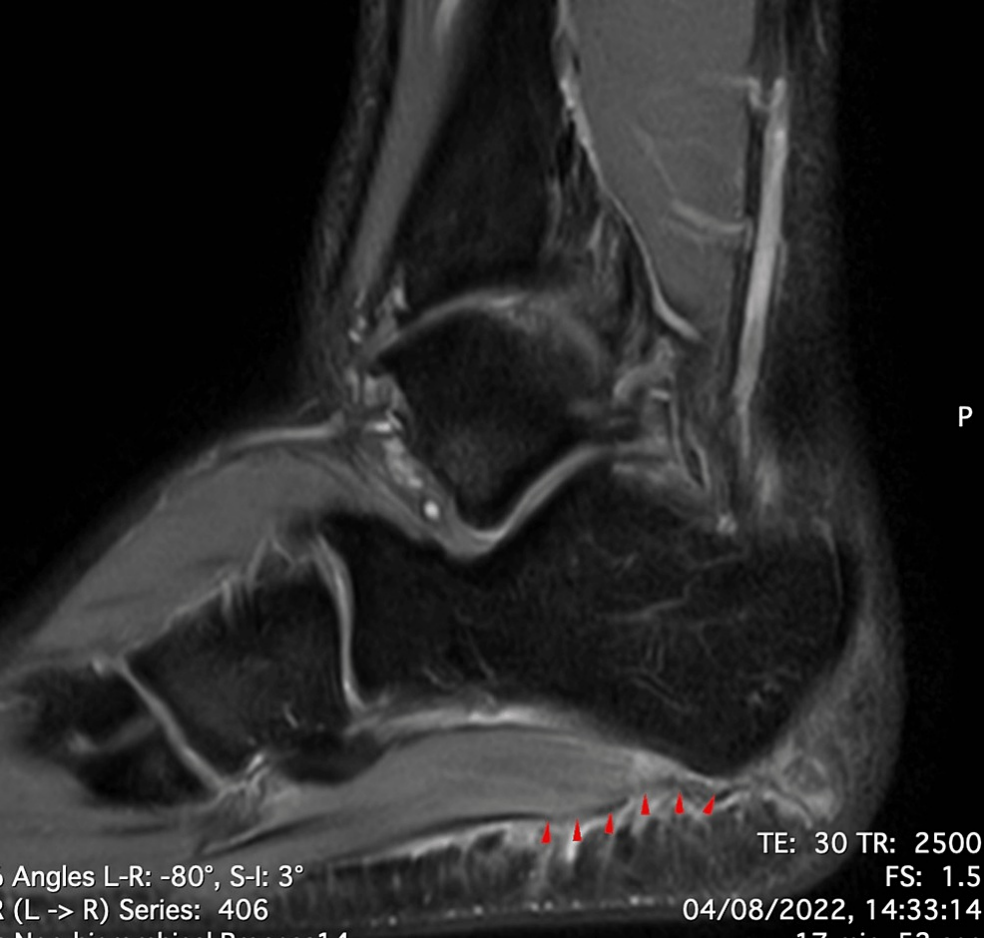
Saggital cut of proton-density MRI. Full-thickness tear of the proximal segment of the lateral band of the plantar fascia, which appears with a bright signal (red arrows).
